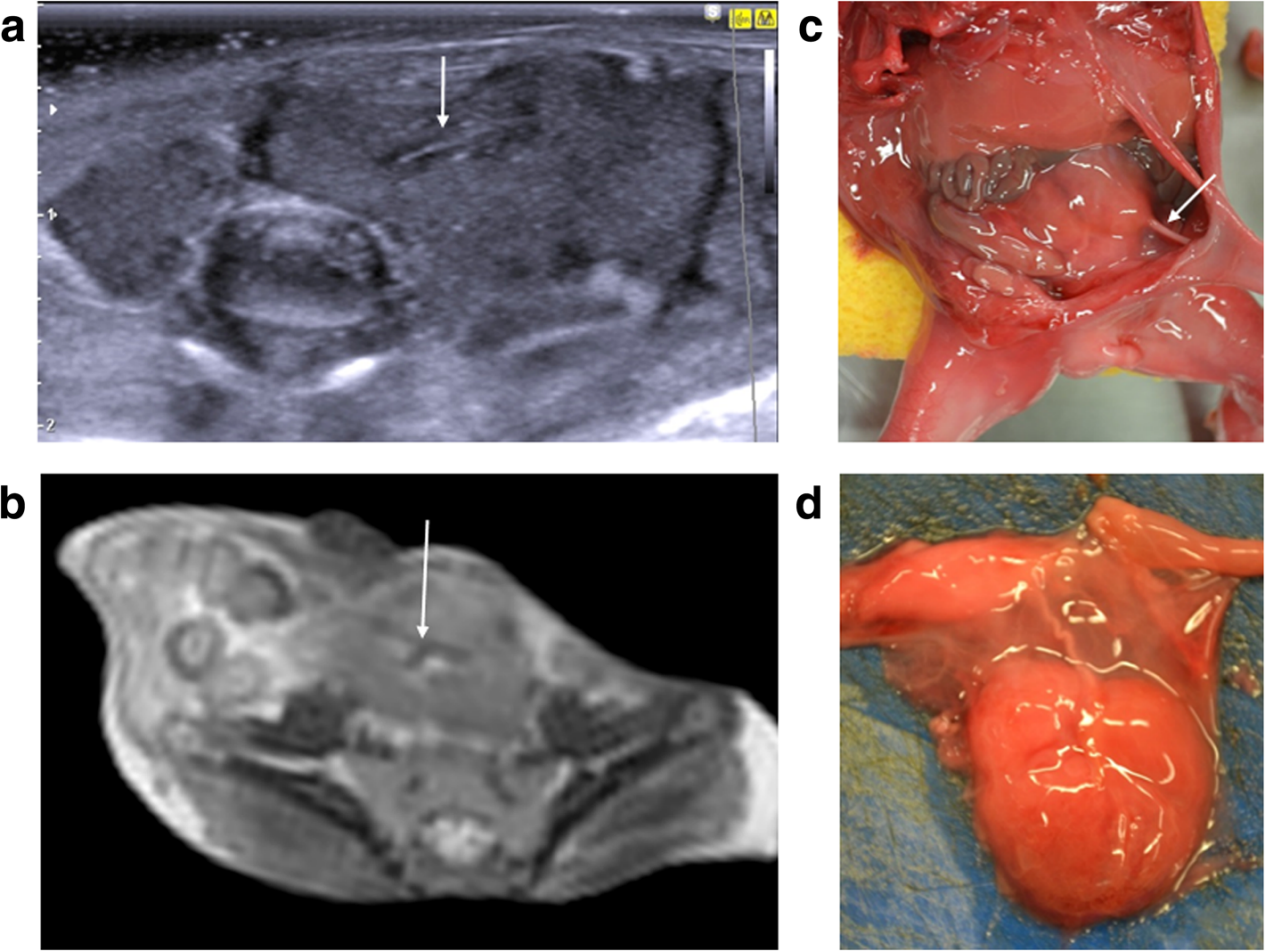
Solitary left pelvic kidney in a foetus at 20 weeks gestation, following termination of pregnancy for presumed renal aplasia. Post-mortem imaging was obtained 3 days after delivery. Transverse post-mortem ultrasound image (a) demonstrates a soft tissue mass in the left iliac fossa which lacks the normal expected renal corticomedullary differentiation but appears to have a demonstrable hilum (solid arrow). The same finding is demonstrated on the corresponding post-mortem T2 weighted MRI study, obtained in axial plane (b) and also on photographs obtained at autopsy (c), acquired after careful dissection of the anterior abdominal wall. The extracted soft tissue mass (d) was confirmed to be renal in origin
